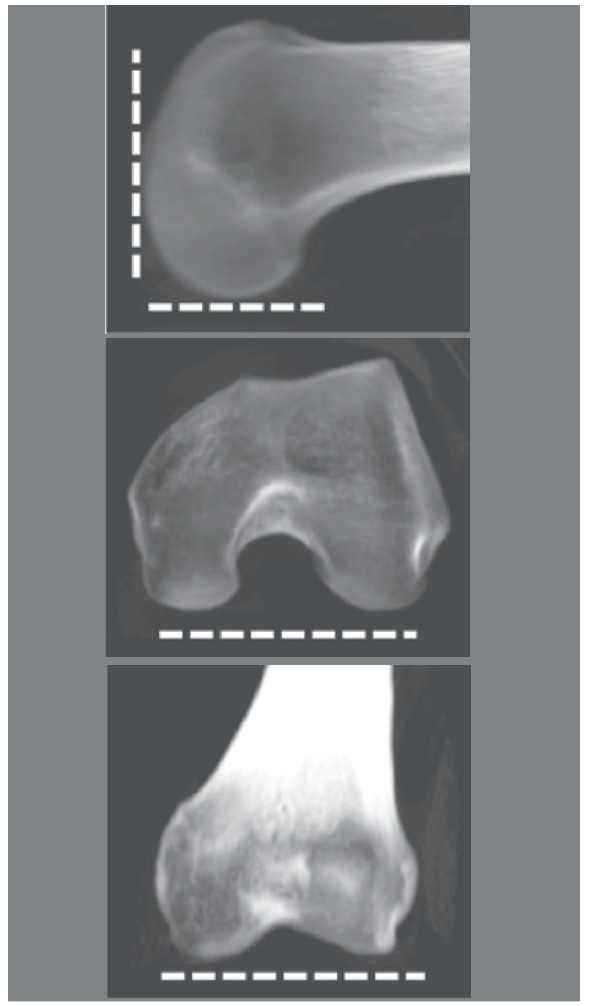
Transparent CT scan: inferior and posterior condyle wall alignment in sagittal, axial and coronal view.
